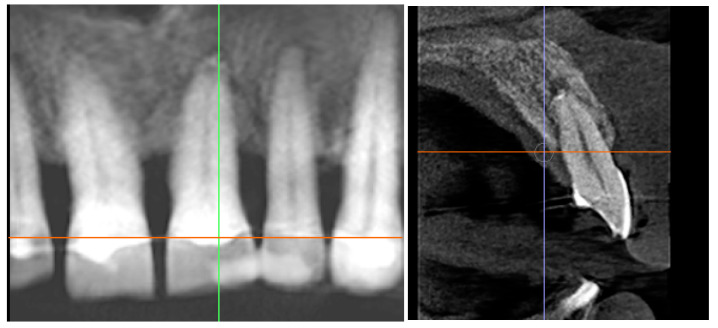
Preoperative CBCT cross-section (baseline).
